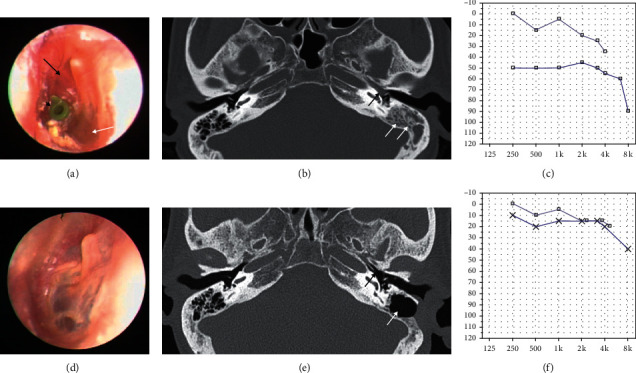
Preoperative (a–c) and postoperative (d–f) clinical findings. (a) Preoperative otoendoscopic examination revealed a pinkish mass-like lesion (black arrow) behind the anterior portion of the tympanic membrane. Middle ear effusion is observed (white arrow) despite previous ventilation tube insertion (black arrow head). (b) Axial view of temporal bone computed tomography (TBCT) demonstrated soft tissue density obstructing the bony portion of the Eustachian tube (black arrow) and mastoiditis (white arrows). (c) Pure tone audiometry showed conductive hearing loss with an air-bone gap of 37 dB in the left side. (d) Postoperative otoendoscopic examination revealed normal tympanic membrane. (e) Axial view of TBCT demonstrated a clean mastoid cavity (white arrow) and no residual mass in the bony portion of the Eustachian tube (black arrow). (f) Pure tone audiometry showed that the air-bone gap was reduced to 5 dB in the left side.
